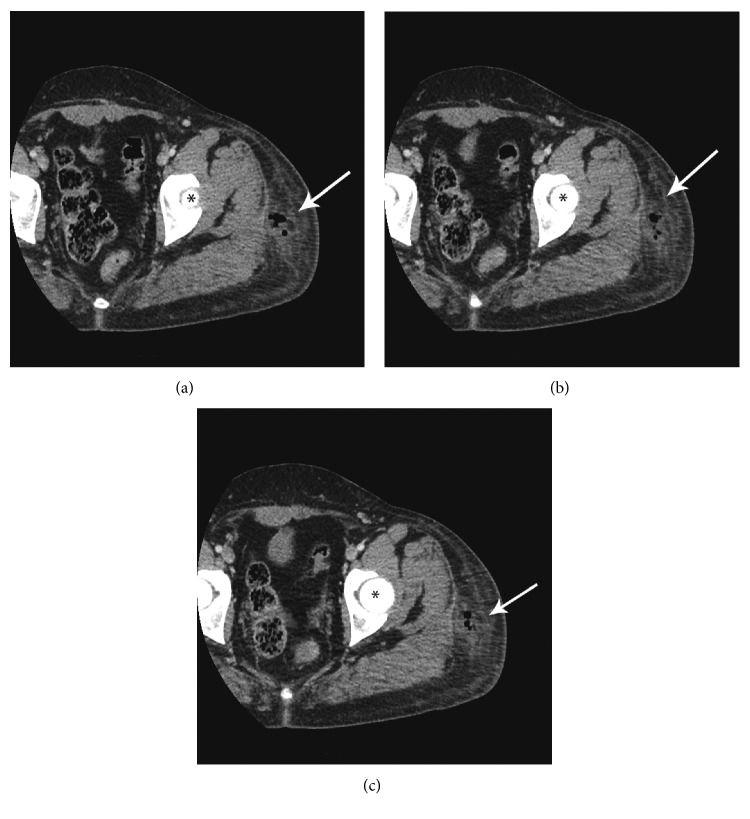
(a, b, c) Three sequential transverse section CT scan images showing the abscess with gas bubbles (arrows); the femoral head is indicated with an asterisk.
